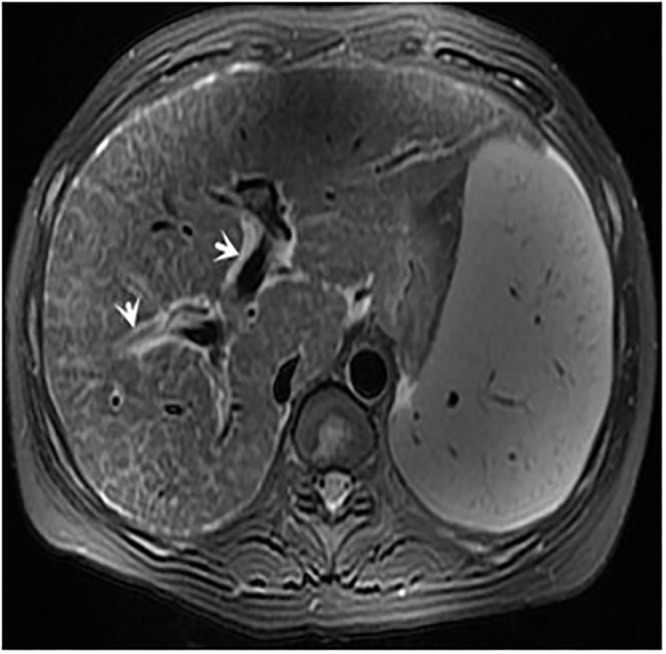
female, 42 years, axial T2-weighted image shows splenomegaly and demonstrates periportal hyperintensity around medium-sized portal triads (*arrows*).
